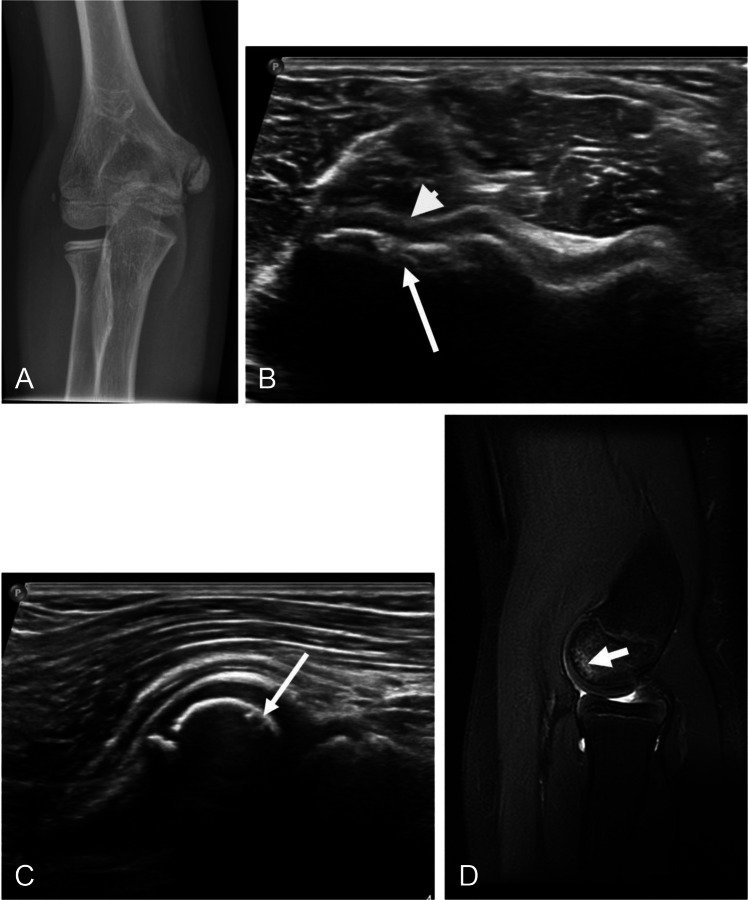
A 10-year-old male baseball player with chronic elbow pain. A Frontal radiograph of the elbow appears normal. B Axial and C long-axis ultrasound images demonstrate focal subchondral irregularity of the capitellum (*arrows*). Intact overlying hypoechoic hyaline articular cartilage (*arrowhead*). D Sagittal short tau inversion recovery MR image confirms subchondral marrow signal abnormality consistent with osteochondritis dissecans (*arrow*)
